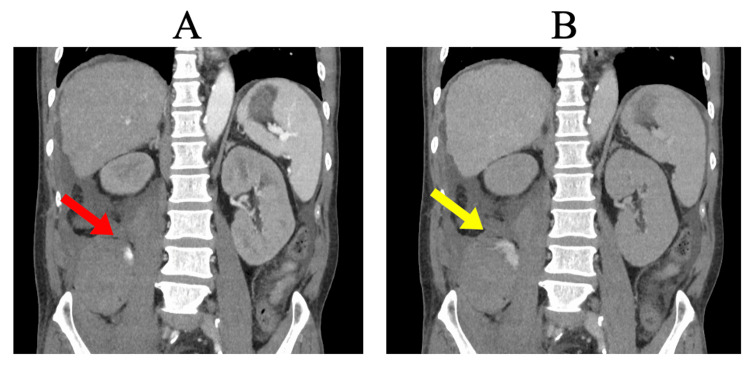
Contrast-enhanced abdominal CT six days after renal biopsy (A) Arterial phase (40 s post-contrast) showing active retroperitoneal bleeding from the right lumbar artery (red arrow). (B) Delayed phase (100 s post-contrast) showing persistent contrast extravasation in the retroperitoneum (yellow arrow). CT, computed tomography.
